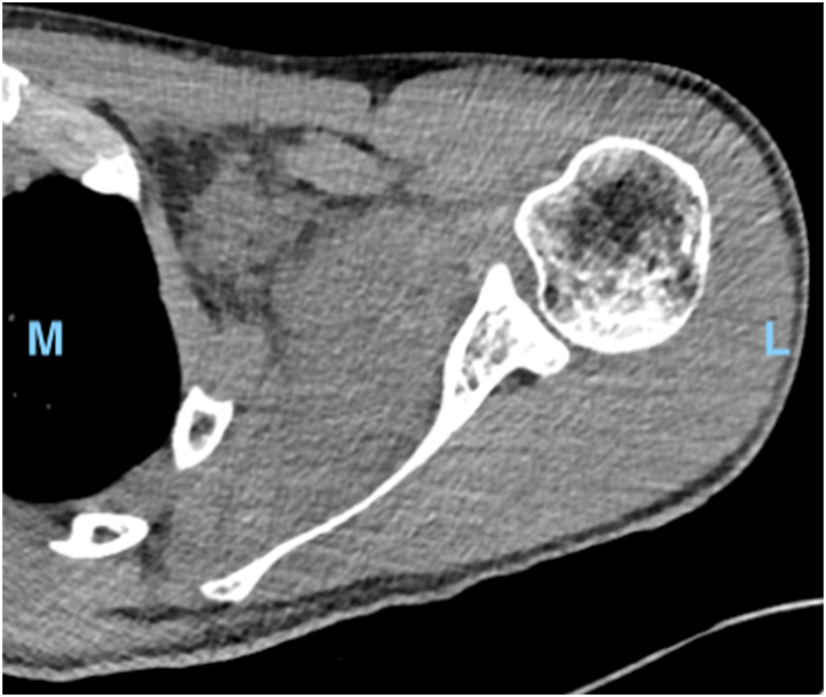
Transverse computed tomography (CT) scan of left shoulder. L, lateral. M, medial.
